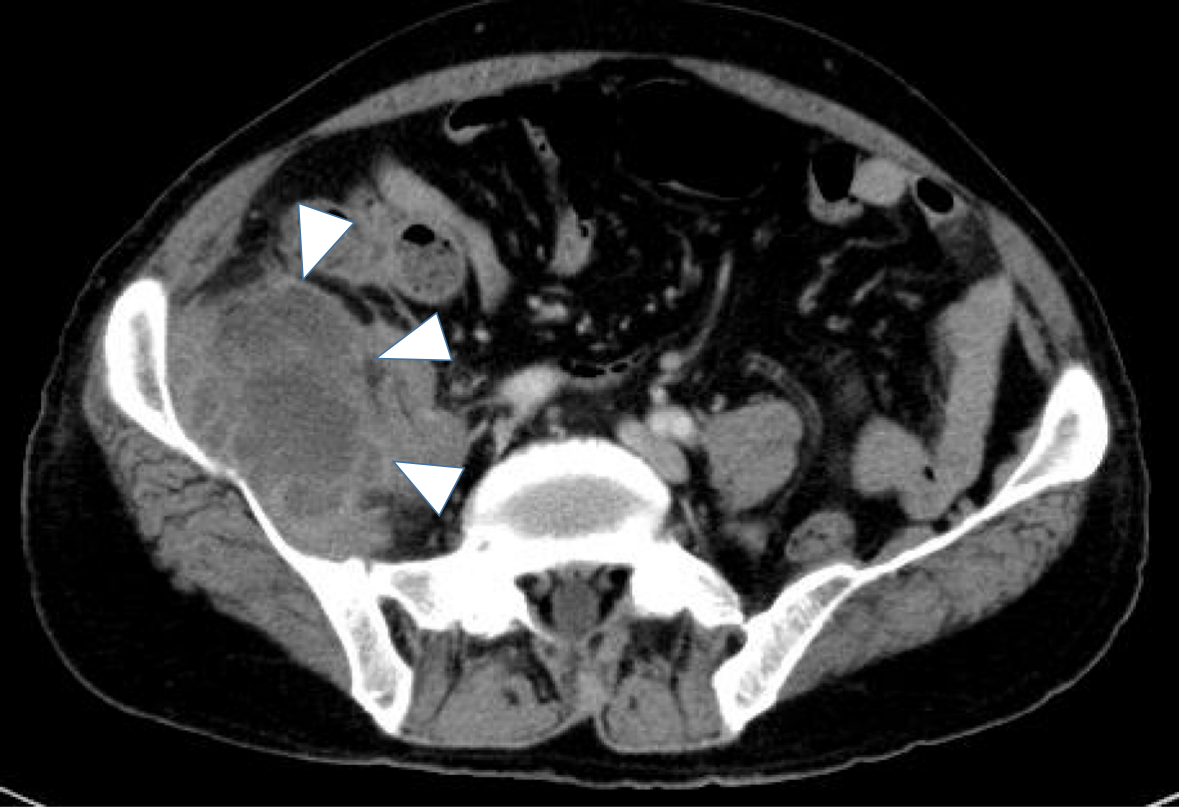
CT image. Enhanced computed tomography revealed a heterogeneous contrast-enhancing mass lesion measured to be 55 × 50 mm in size at the lower right abdomen (white arrow)
